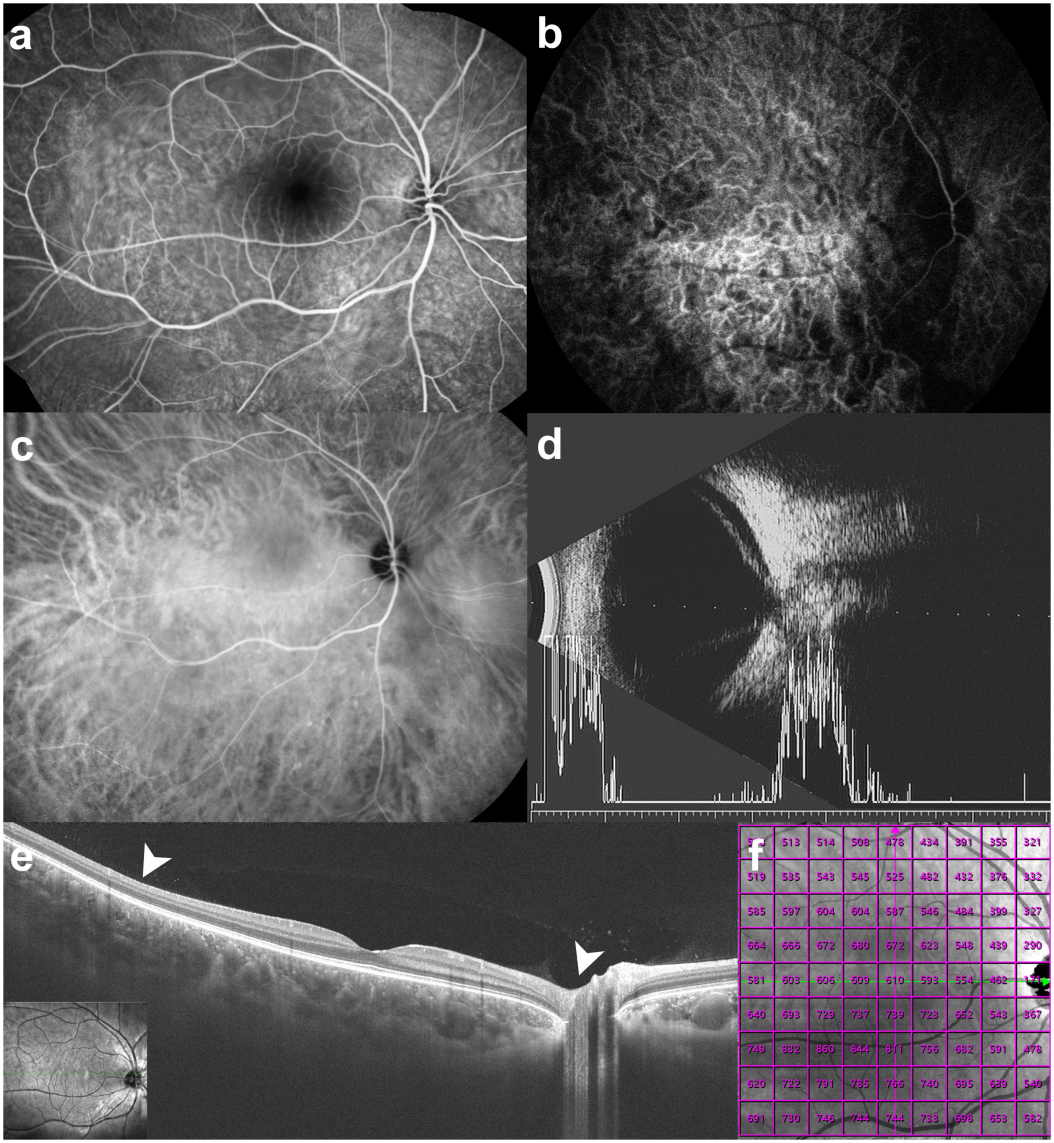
Multimodal imaging examination images of the eyes. (a) Fluorescein fundus angiography of the right eye shows diffuse hyperfluorescence in the choroidal hemangioma area (at 6 min and 4 s after injection). (b) Indocyanine green angiography shows immediate filling of the choroidal hemangioma at 1 s after injection. (c) The lesion displays a coarse, “spongy” vascular pattern with well-demarcated, homogeneous hyperfluorescence at 47 s after injection. (d) B-scan ultrasonography of the left eye reveals a funnel-shaped excavation of the posterior fundus centered on the optic disc, with an irregular, hypoechoic area within it. A V-shaped band of moderate to low echogenicity surrounds the disc, which is highly suggestive of exudative retinal detachment. (e) Optical coherence tomography reveals marked thickening of the choroid with homogeneous, low-reflective luminal spaces. (f) Quantitative analysis confirms a significant increase in choroidal thickness in the area indicated by the two arrowheads in panel e.
